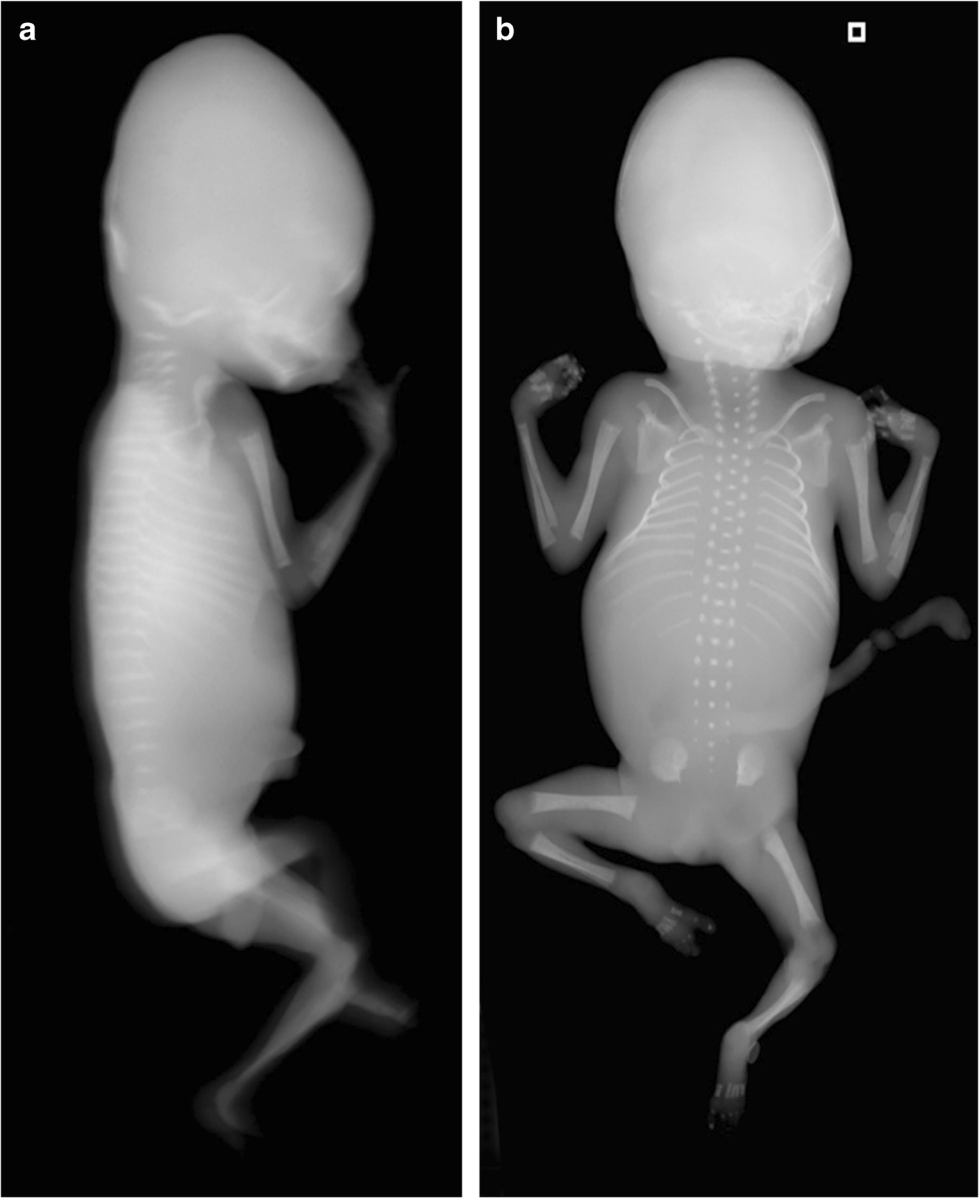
Normal ossification of the fetal skeleton of a male fetus at 14 weeks’ gestation. a,b Radiographs in lateral (a) and anteroposterior (b) projections show ossification of all vertebral bodies, which is in contrast with characteristics shown in the 11-week fetus in Fig. 1; however, there remains absent ossification of the skull vault, much of the skull base, and the ischia and pubic bones
